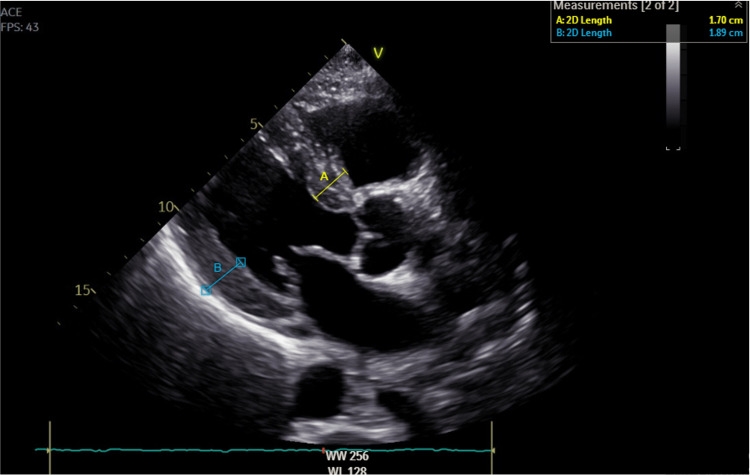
Parasternal long-axis view echocardiography revealing severe concentric left ventricular hypertrophy (posterior wall 19 mm), thickened interventricular septum (interventricular septum 17 mm), and atrioventricular valves. A = interventricular septum; B = posterior wall
